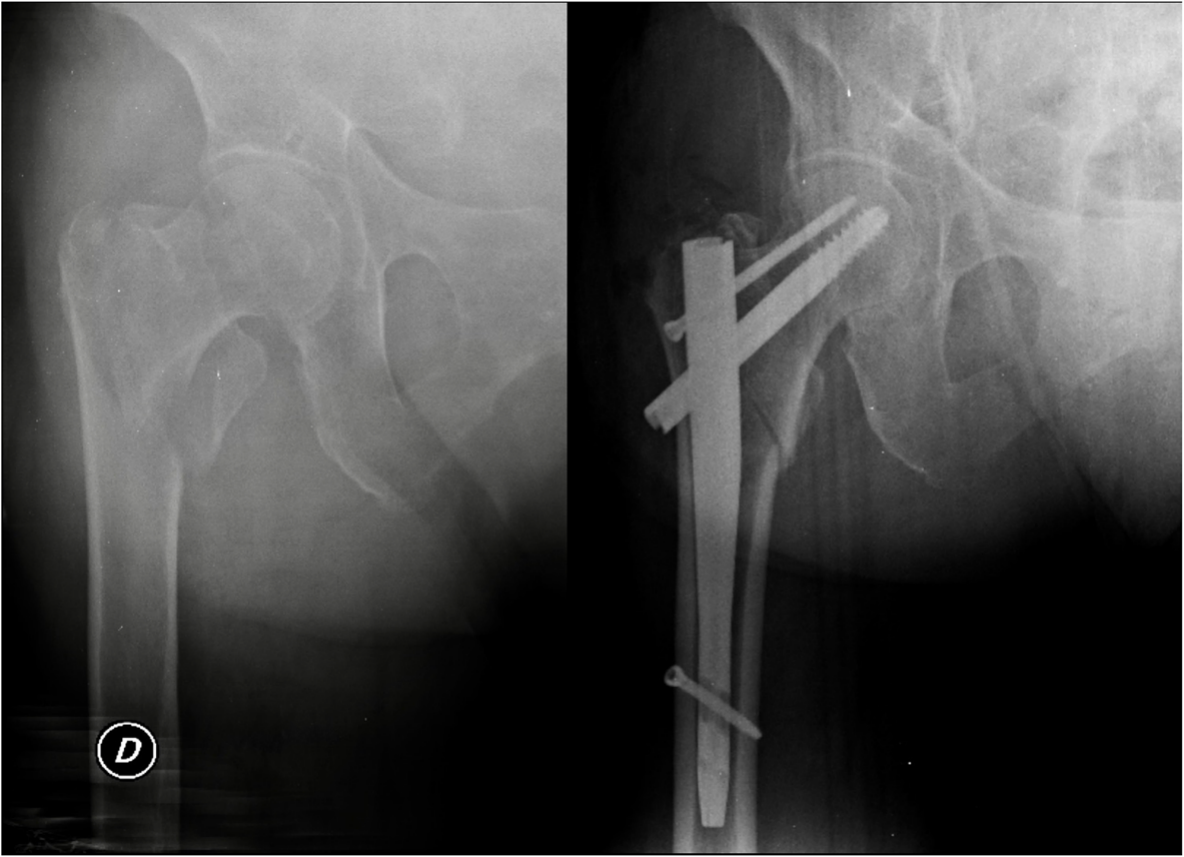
Pre-operative and post-operative x-rays of a patient with a pertrochanteric fracture (31.A2, pertrochanteric multifragmentary) treated with Elos® nail
